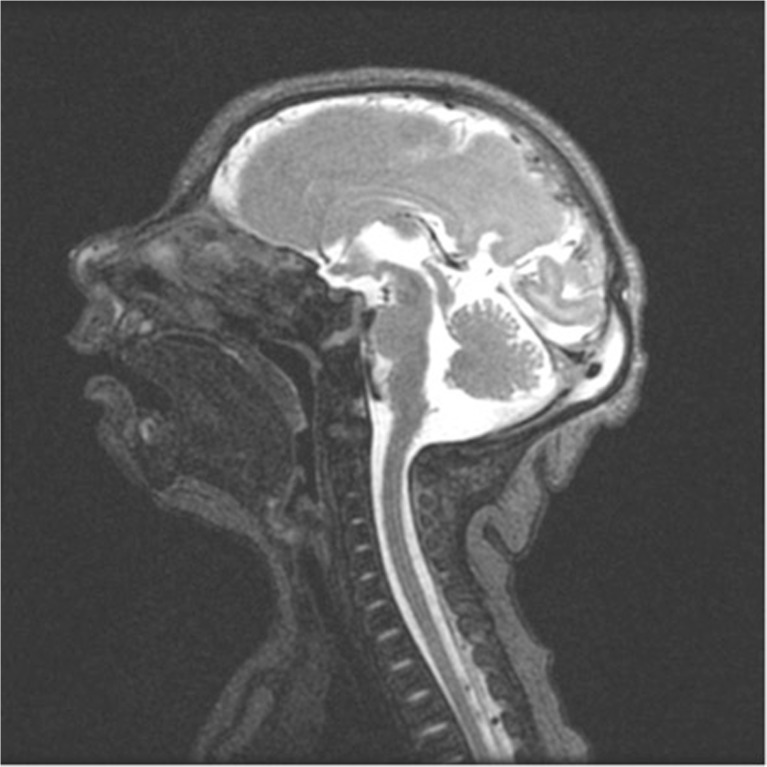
Skull MRI with craniofacial disproportion, increased subarachnoid space, and corpus callosum hypoplasia. The brainstem, cerebellum, and spinal cord are preserved
